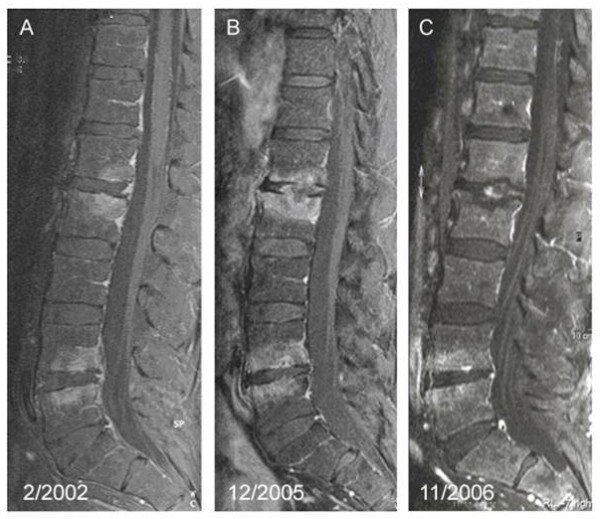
MRI alterations during follow-up. Contrast-enhanced, T1 weighted, fat saturated MRI demonstrating progression of two spondylitic lesions with the onset of clear erosions in the L1/L2 segment during treatment with etanercept, a TNF-α blocking agent between years 2002 and 2005. After cessation of the TNF-α blocker and initiation of antibiotic treatment, the multisegmental spondylitis regressed (year 2006).
